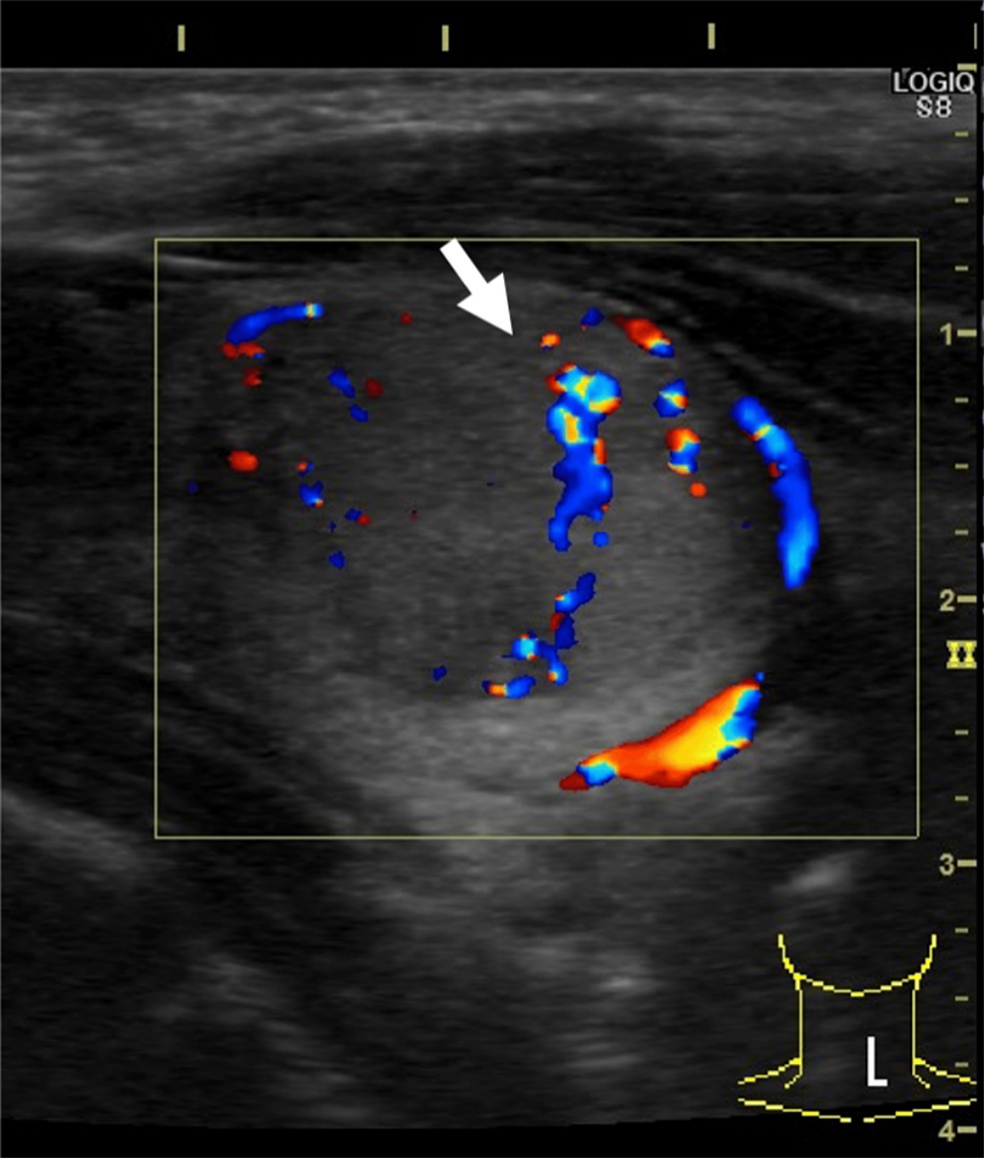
Ultrasound Blood flow inside the tumor and well-defined borders
